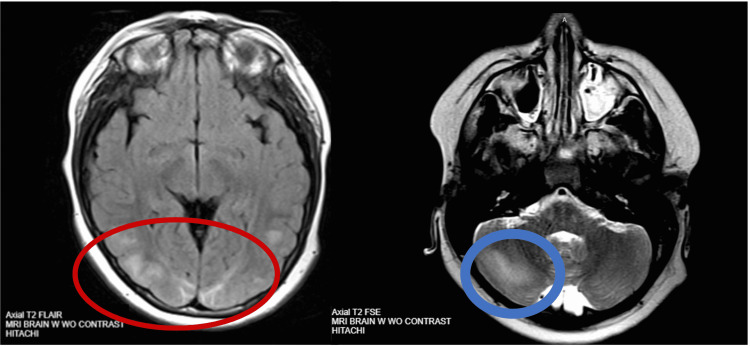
Brain T2 magnetic resonance imaging without contrast. Large regions of abnormal signal in both cerebral hemispheres, predominantly posteriorly (red circle) and in the right cerebellar hemisphere (blue circle), suggestive of PRES. FSE: fast spin echo; FLAIR: fluid-attenuated inversion recovery; PRES: posterior reversible encephalopathy syndrome
